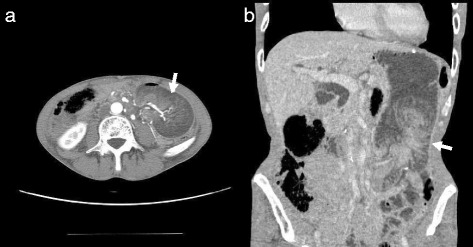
a, b Abdominal computed tomography showing a tumor with a layered structure in the left abdomen (*white arrow*). Intestinal obstruction secondary to intussusception was suspected
